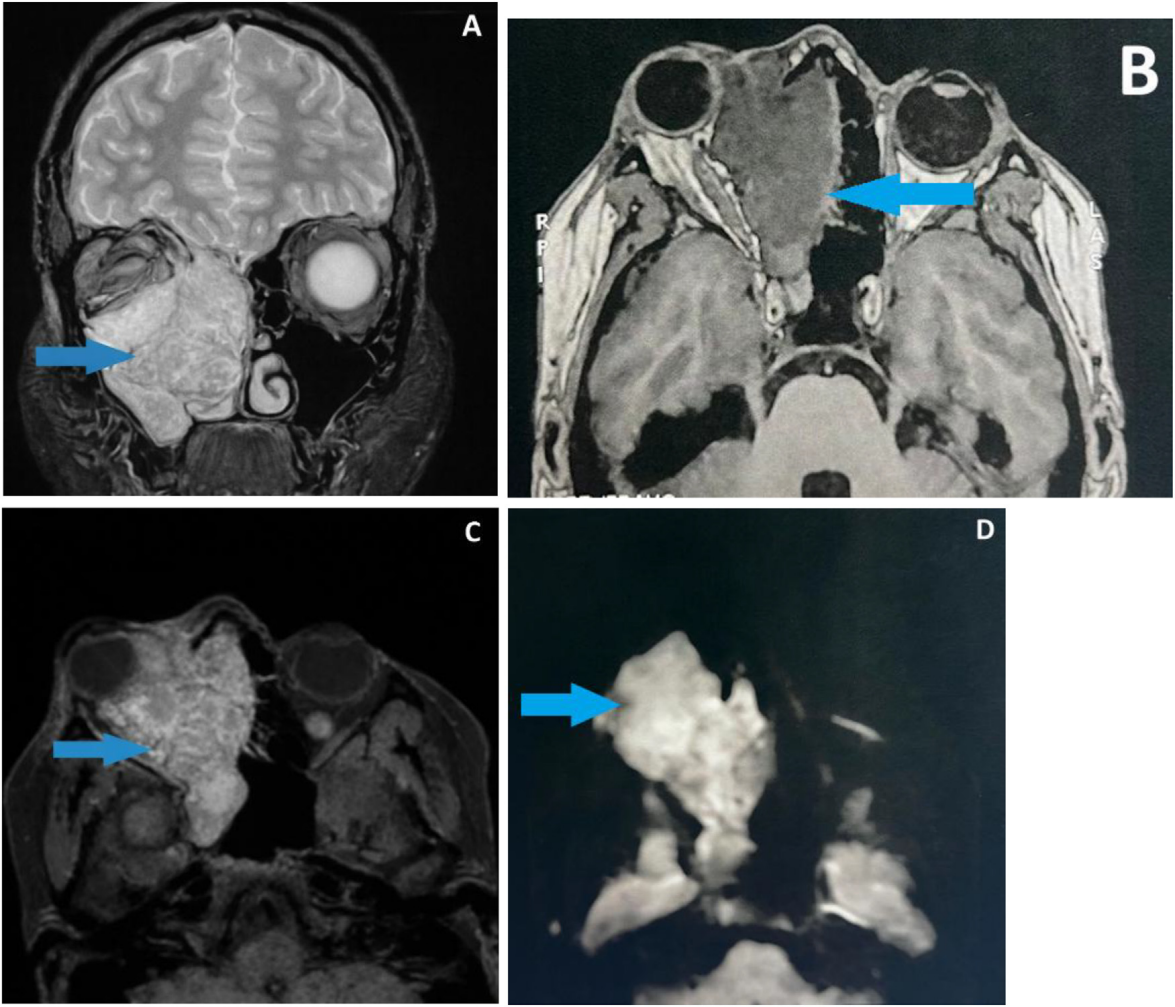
Head and neck MRI demonstrates a right maxillo-orbito-ethmoido-nasal mass (blue arrow) measuring 68 × 60 × 48 mm. The lesion appears isointense on both T2 (A) and T1-weighted (B) sequences, exhibits heterogeneous enhancement following gadolinium administration (C), and shows restricted diffusion with high signal intensity on DWI (D). The mass exerts a marked mass effect on the deformed right globe, resulting in grade III proptosis, and extends superiorly into the right basifrontal region.
